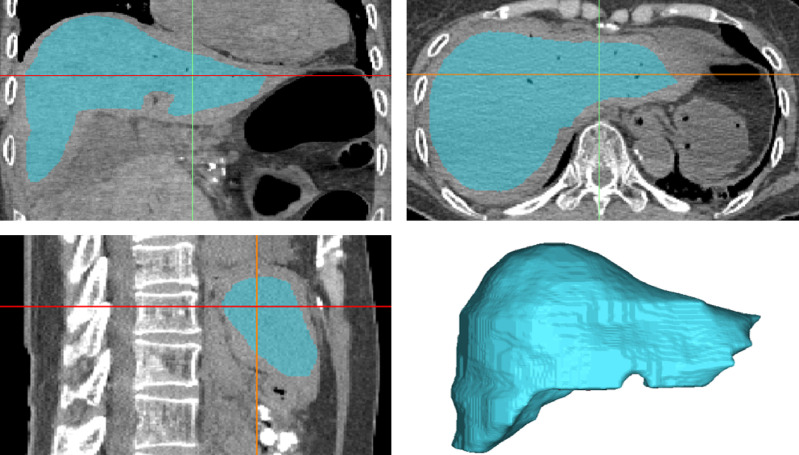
Three-dimensional reconstruction of the whole liver on postmortem computed tomography using Mimics.
